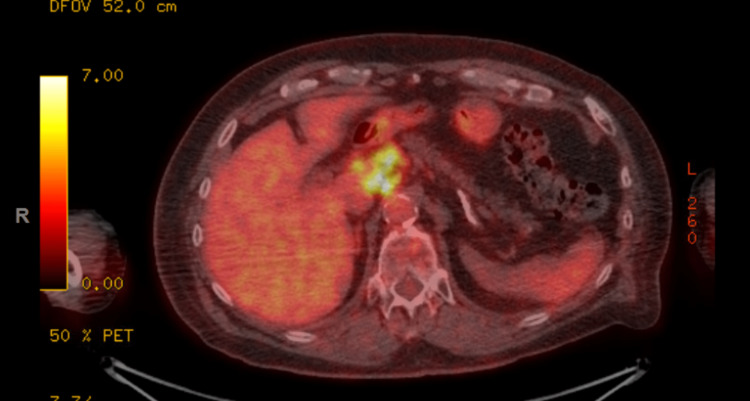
FDG-avid lymphadenopathy in the upper abdomen FDG: 18F-fluorodeoxyglucose
